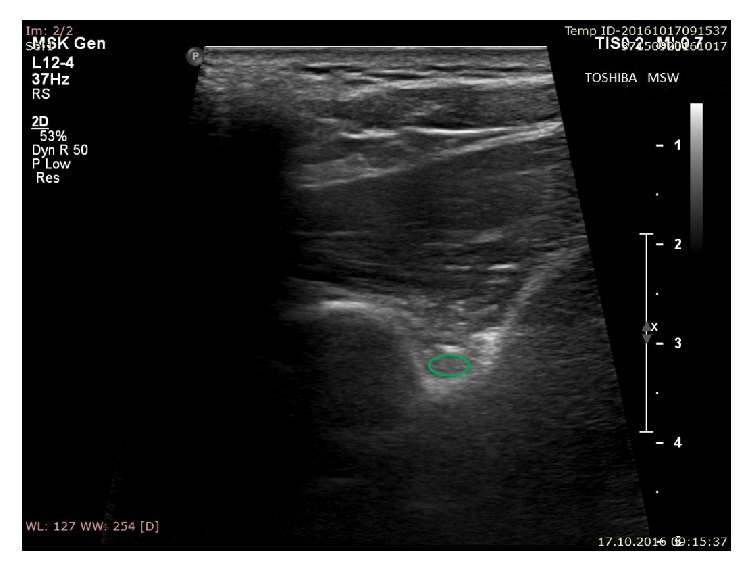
Ultrasonographic investigation of the suprascapular notch region. Green: suprascapular nerve.
